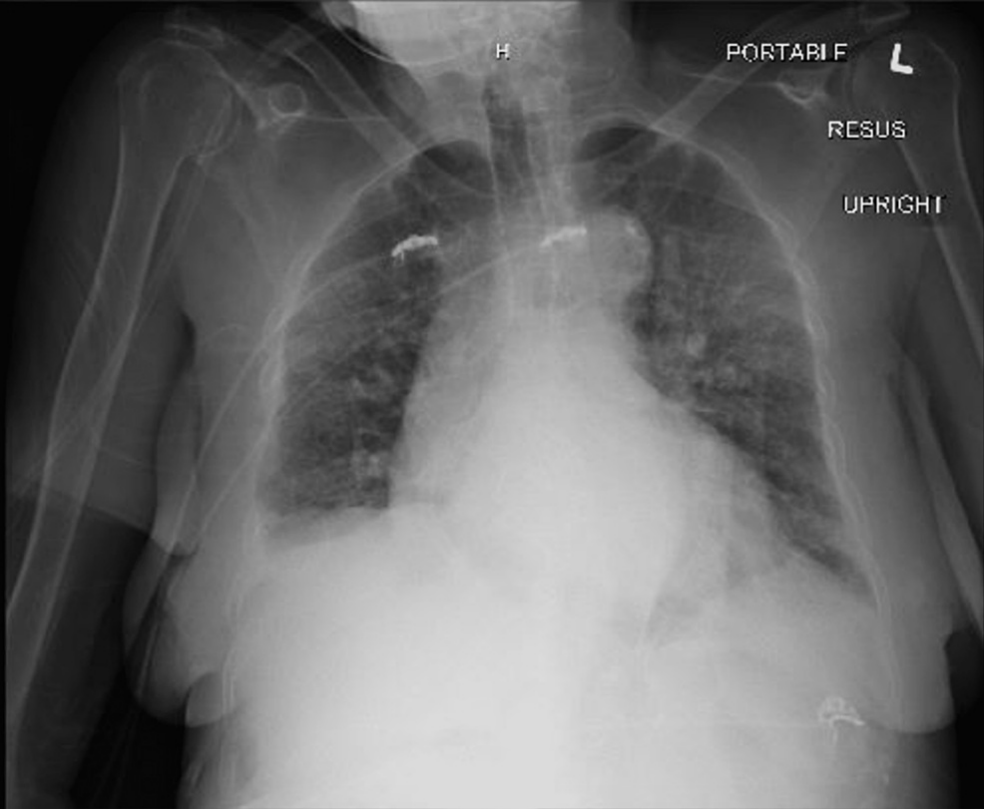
Chest X-ray on admission showing left perihilar hazy, ill-defined opacity with previous right lower lobe lobectomy
